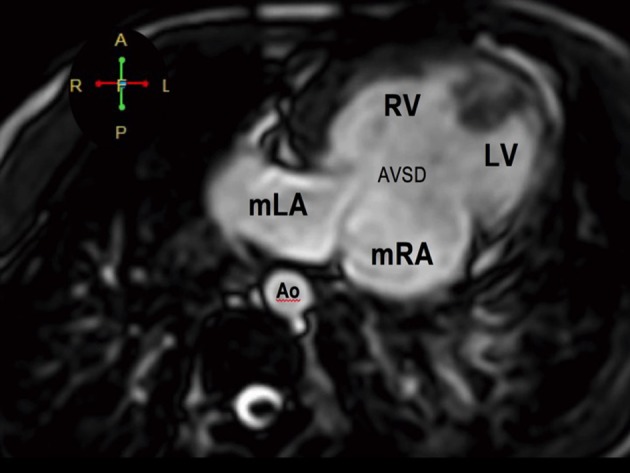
Atrioventricular discordance on cardiac MRI. The morphologic LA drains into the morphologic RV while the morphologic RA drains into the morphologic LV. There is a complete atrioventricular septal defect (AVSD).
